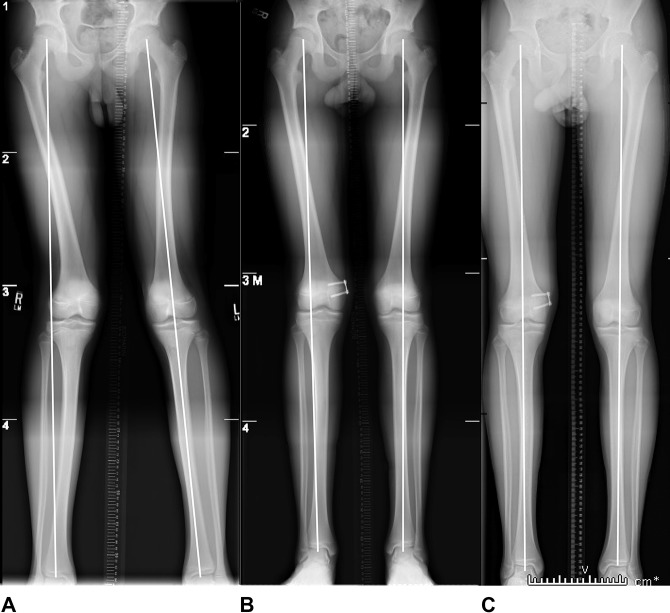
Serial standing alignment radiographs of an adolescent boy (patient #3) with lateral distal femoral hemiphyseal arrest treated with guided growth until resolution of his mechanical axis. A, demonstrates the patient's presenting radiographs. B, represents the patient’s radiographs 9 months after starting guided growth with a medial tension band. C, represents the patient 14 months after initiation of guided growth with restoration of his mechanical axis.
